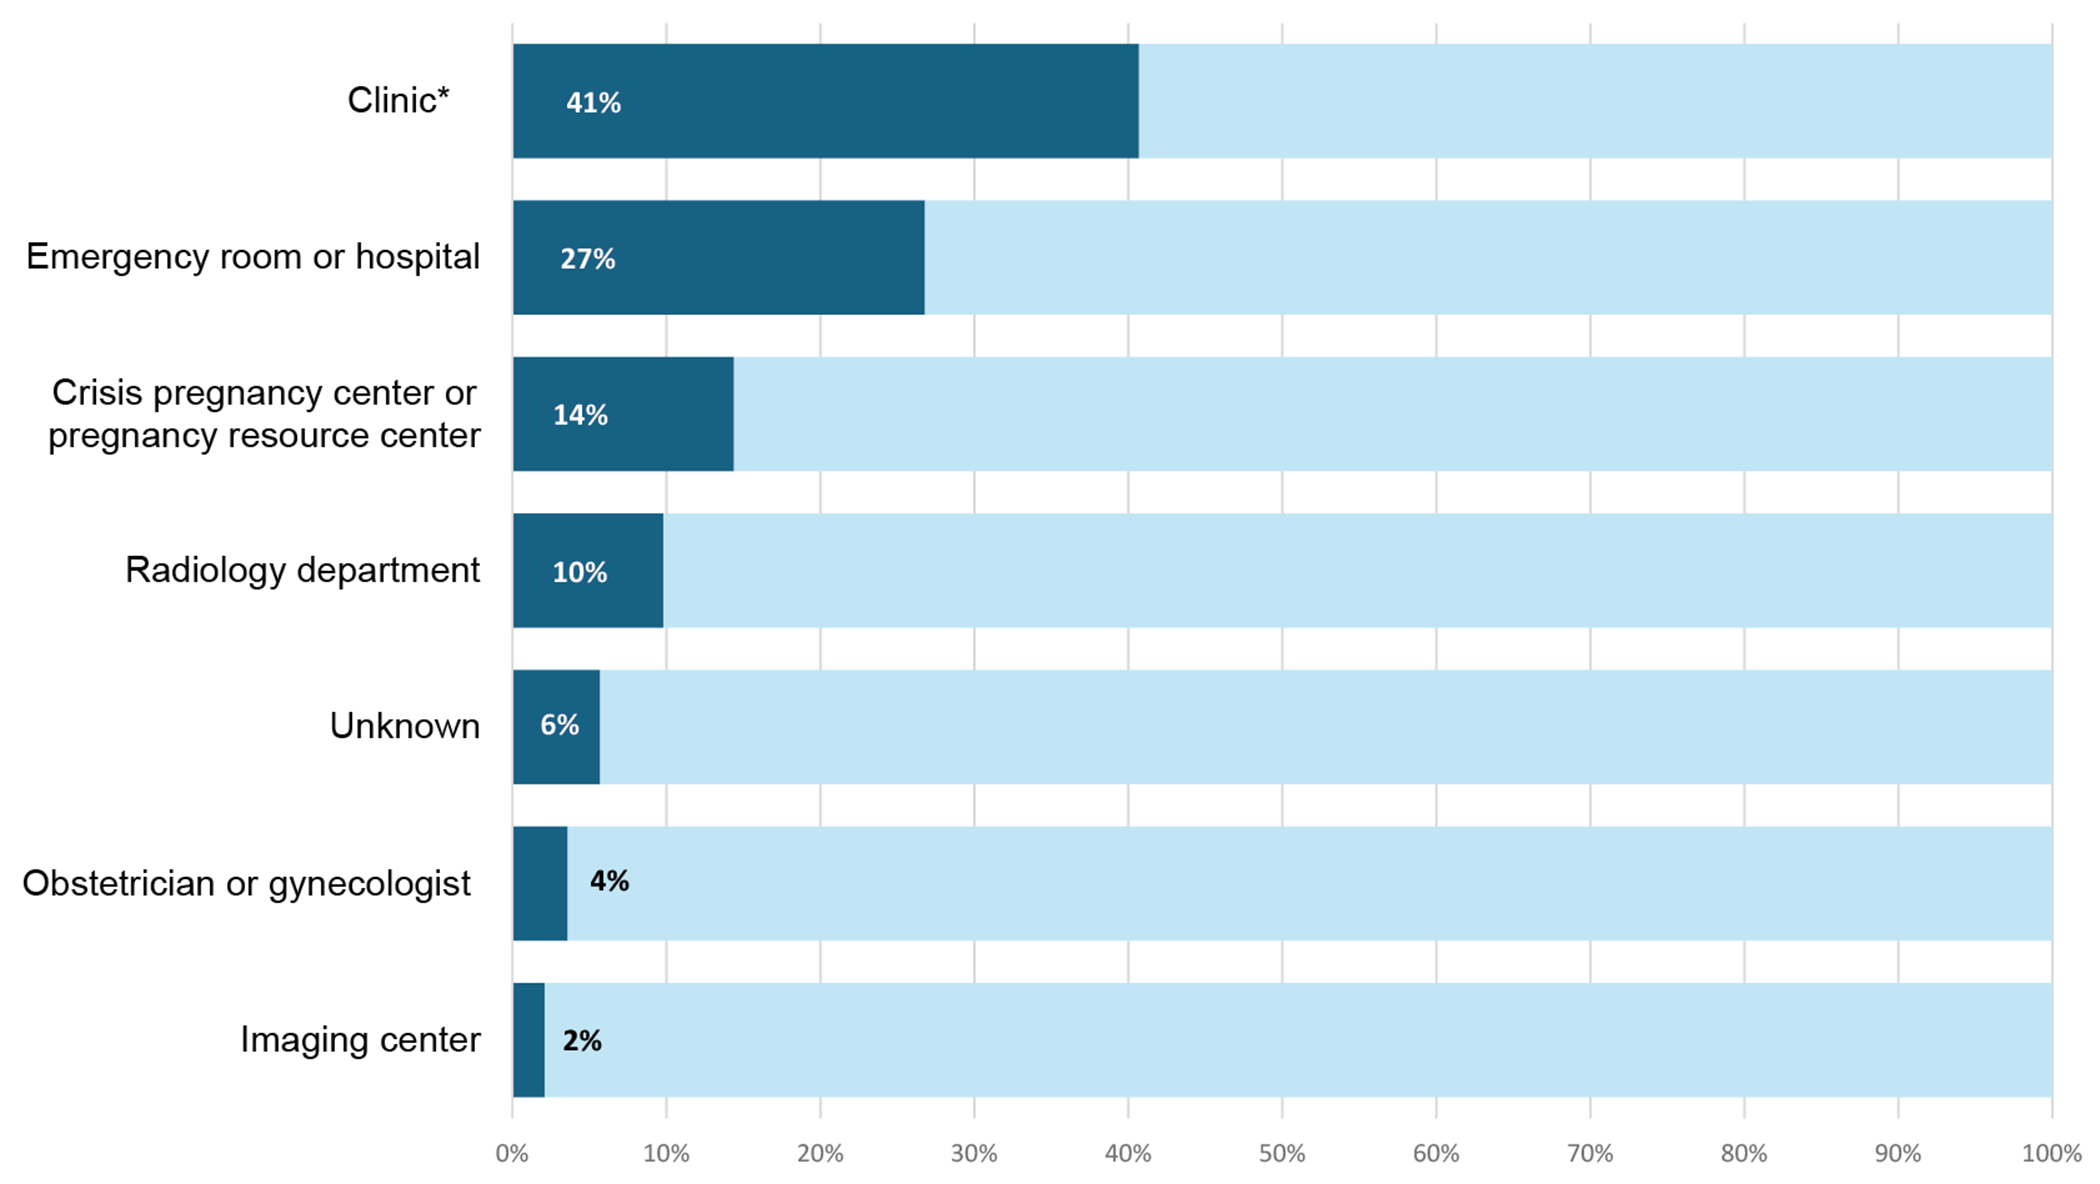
Locations where telehealth abortion patients obtained ultrasonographic imaging in the California Home Abortion by Telehealth Study from 2021 to 2022. Participants could select more than one response (*n* = 194 for each location). *Clinic includes abortion clinic, family planning clinic, primary care clinic and unspecified clinic
